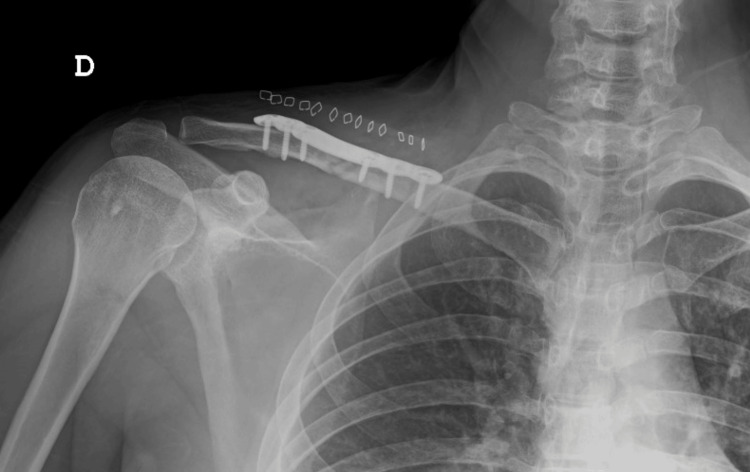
Postoperative X-ray image of right clavicle plate osteosynthesis
